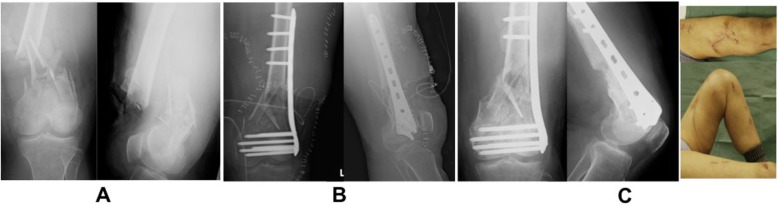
a Radiograph with Gustilo type-IIIA in an 80-year-old woman. b Postoperative radiograph. c Radiograph and CT showed callus formation 2 months postoperatively; left knee range of motion was 0–140°
